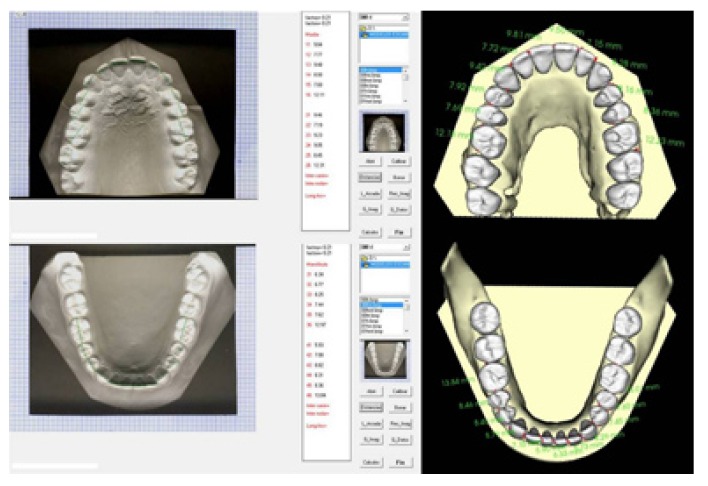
Measurement of mesiodistal tooth-sizes using the Digital Method and CBCT.
